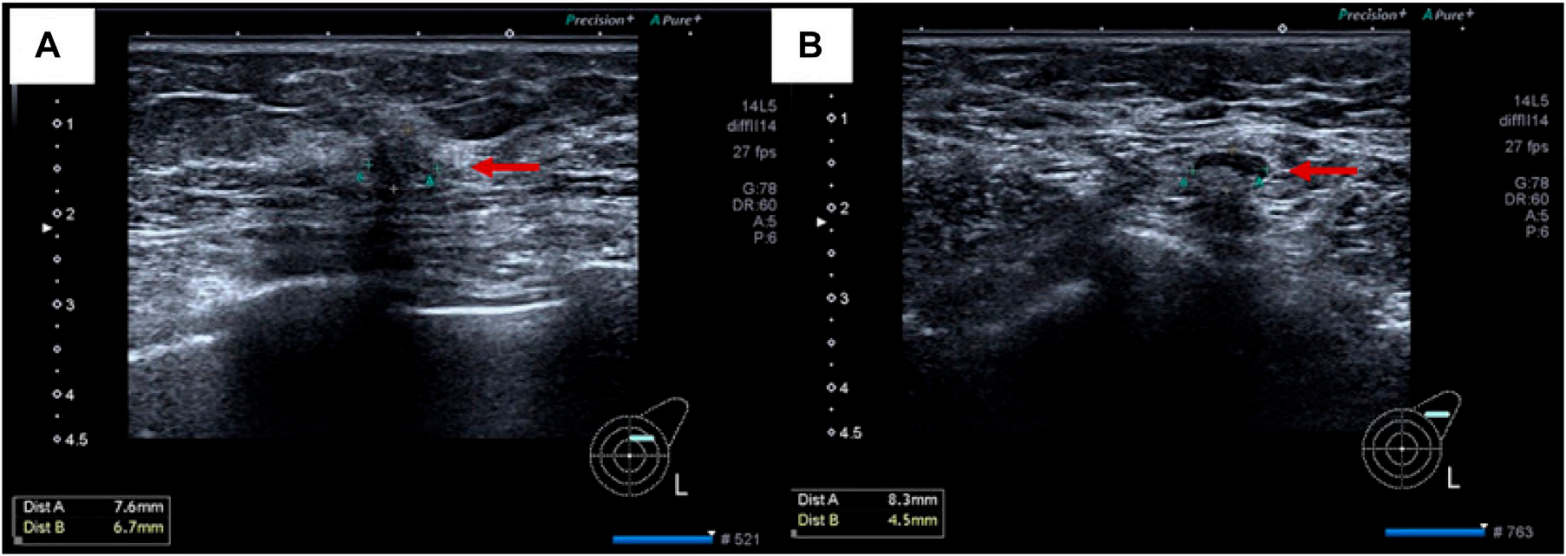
Imaging pictures of the patient at admission. (A) breast ultrasound; (B) lymph node ultrasound.
